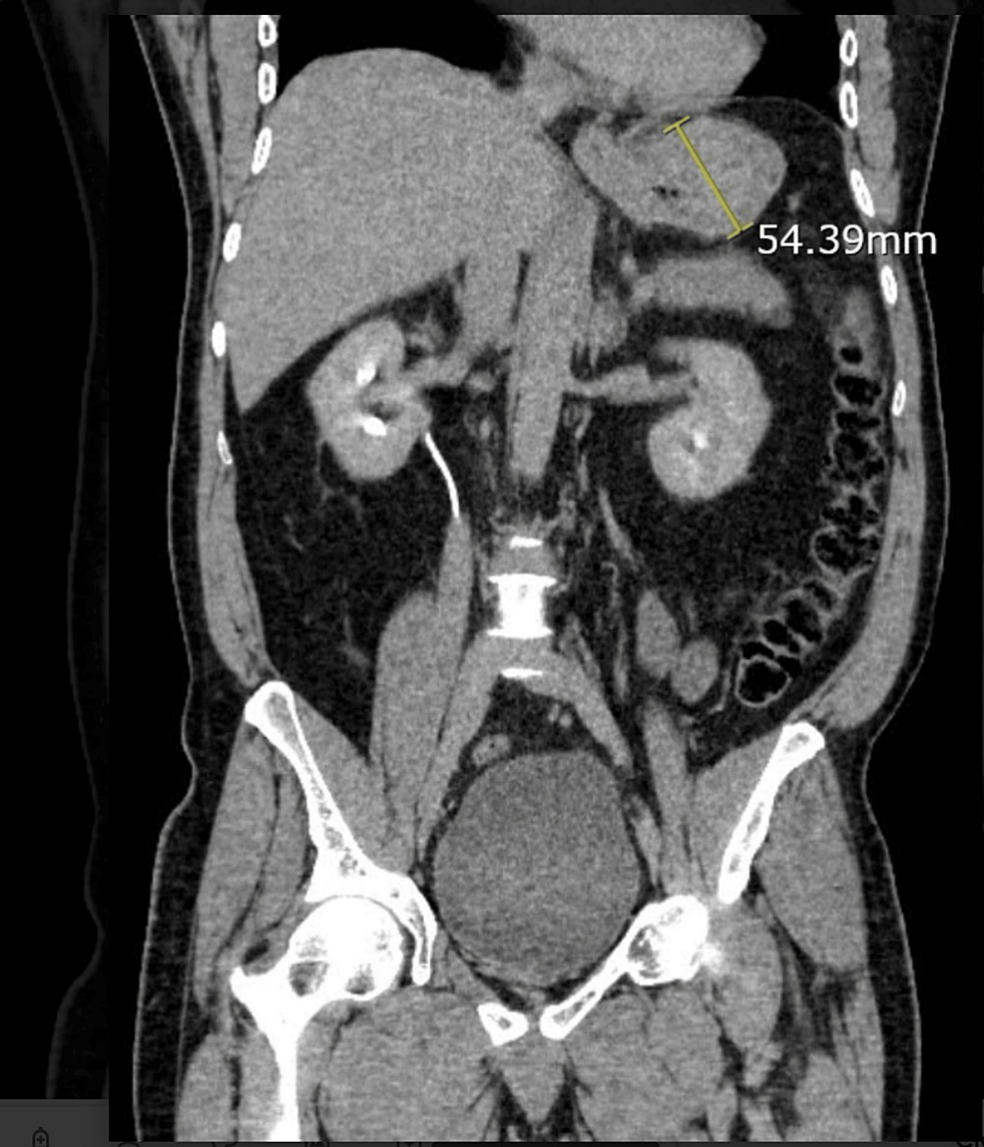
CT of the abdomen and pelvis (coronal section) demonstrating a 5 cm solid mass in the gastric cardia with distal esophageal involvement.
